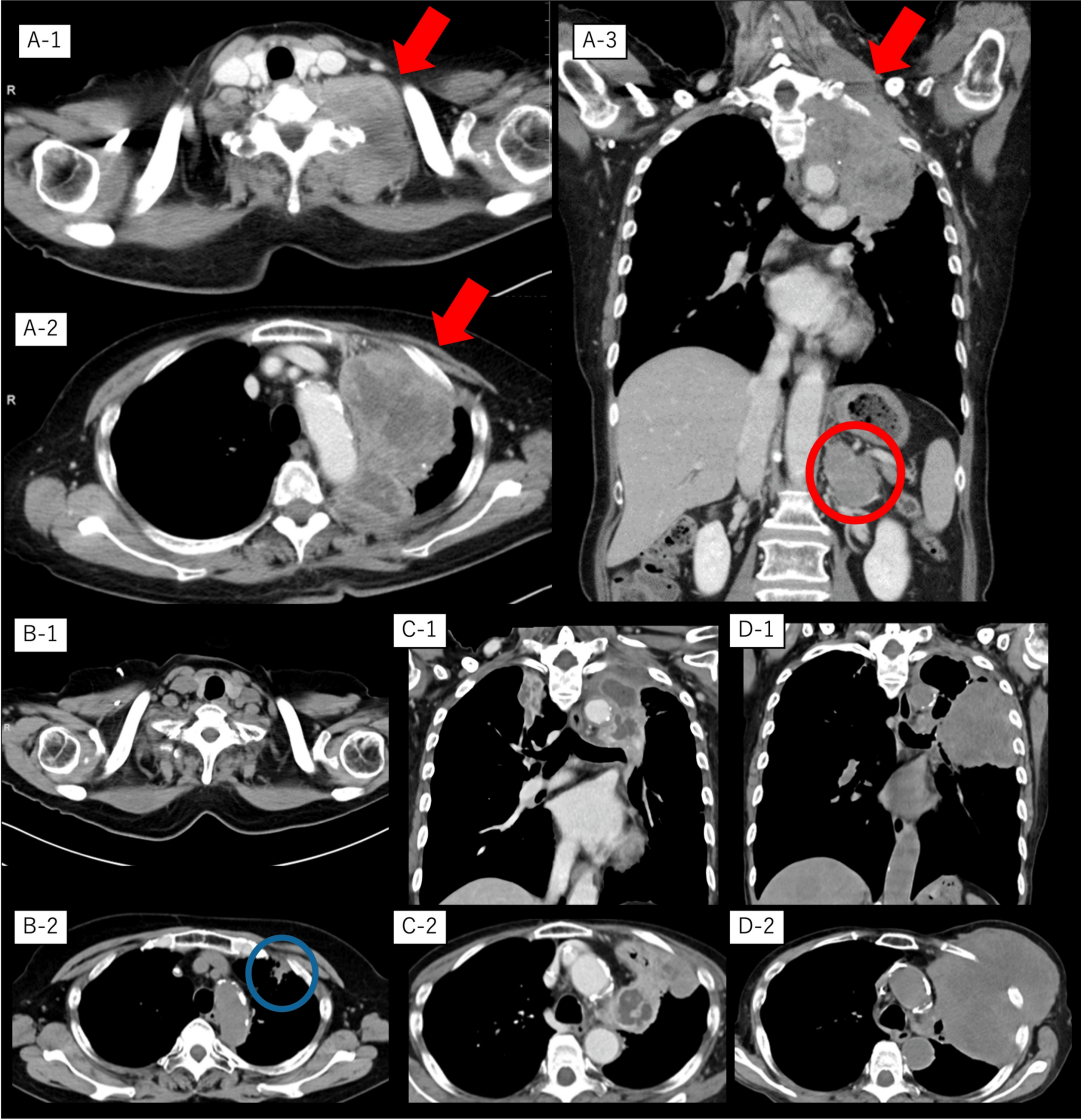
Computed tomography images of the patient during the clinical course Contrast-enhanced computed tomography images at hospitalization (A-1, A-2, and A-3), after six cycles of R-CHOP (rituximab, doxorubicin, cyclophosphamide, vincristine, and prednisolone) therapy (B-1 and B-2), at relapse of lymphomatoid granulomatosis (C-1 and C-2), and just before the patient passed away (D-1 and D-2). Red arrows indicate the primary lesion from the left lung apex to the neck; the red circle indicates a metastatic lesion in the left adrenal gland. A transbronchial lung biopsy performed on the residual lesions showed organizing pneumonia (blue circle).
